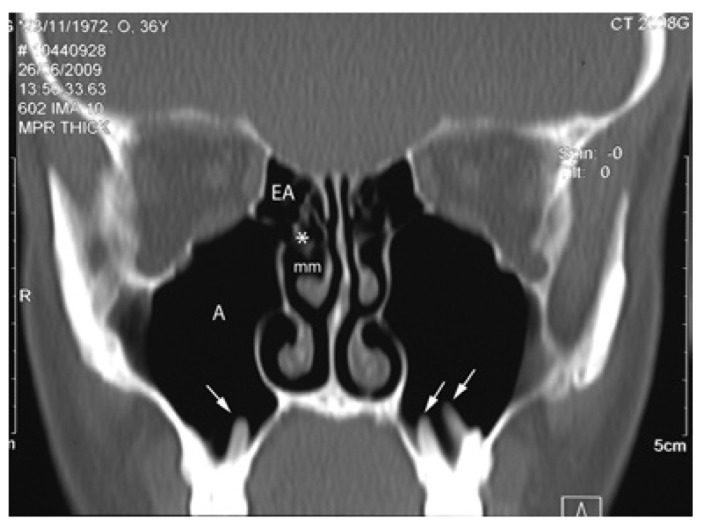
CT scan (coronal plane) of the maxillary and anterior ethmoid area corresponding to a healthy adult. The drainage ostium of the maxillary sinus (A) and those of the anterior ethmoid sinuses (EA), are located in close vicinity (*) in the middle meatus (mm). This image shows a close relationship of the dental roots with the maxillary sinus, which projecting into de antrum (arrows).
